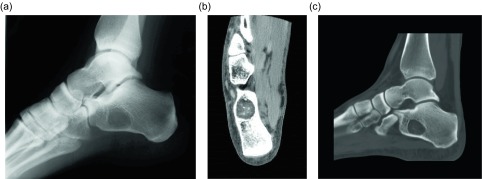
Intraosseous lipoma: 25-year-old female with ankle pain. Lateral radiograph (a) of the right ankle reveal a radiolucent lesion in the anterior portion of the calcaneus with a thin sclerotic border. Axial (b) and sagittal (c) non-contrast CT images again demonstrate the well-circumscribed lesion in the anterior calcaneus with thin sclerotic rim. These images demonstrate the fat attenuation within the periphery of the lesion with central soft tissue density and calcification.
